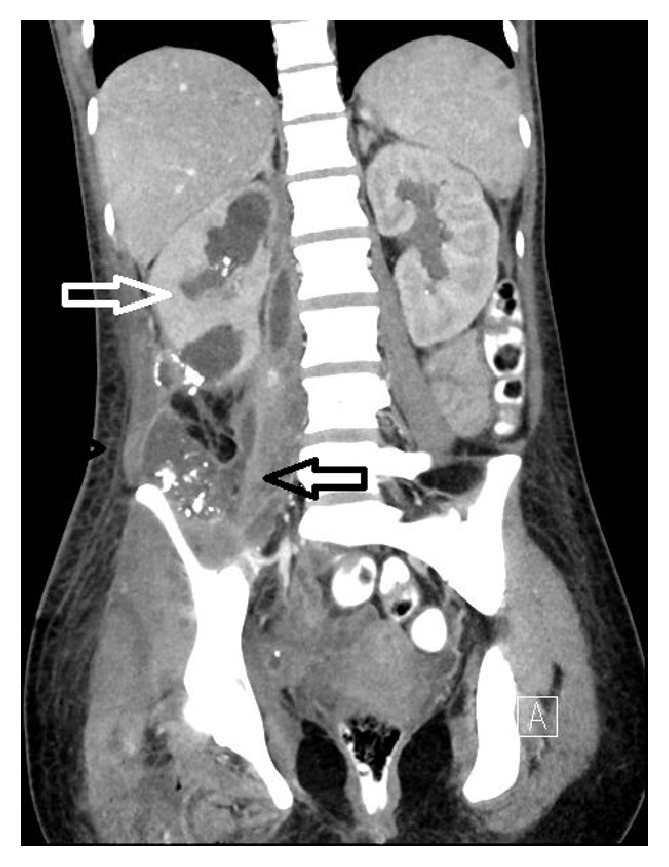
Computed tomography shows a completely disrupted right kidney with multiple stones (white arrow) and a large retroperitoneal abscess (black arrow).
